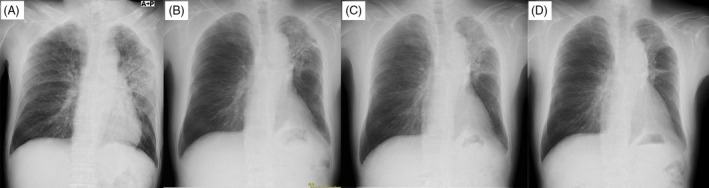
(A) Chest radiography scan obtained at the onset of radiation pneumonitis in April 2020. (B) Chest radiography scan obtained in July 2020. Radiation pneumonitis was improved by prednisolone. (C) Chest radiography scan obtained when the patient was started on dabrafenib and trametinib combination therapy in August 2020. (D) Chest radiography scan obtained in May 2021. As of 35 weeks after initiation of dabrafenib and trametinib combination therapy, the lung tumour shrinkage was maintained without worsening of interstitial pneumonitis.
